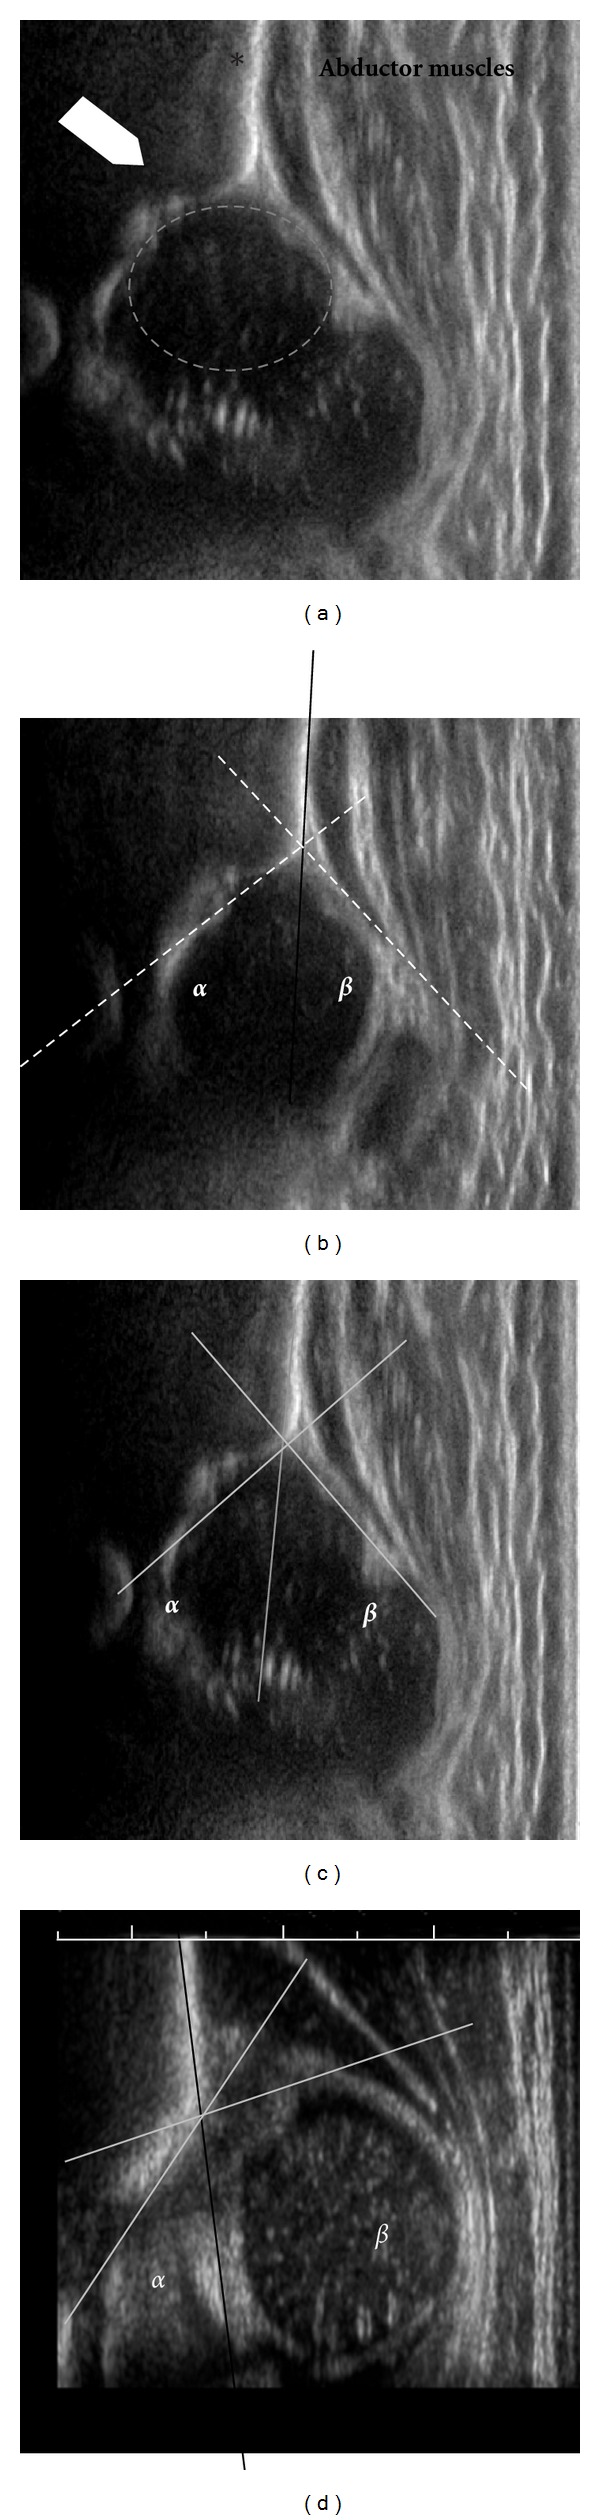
Ultrasound neonatal hip examination. (a) A representative longitudinal ultrasound image of a normal neonatal hip. The ilium is marked by the asterisk, the bony acetabular roof by the large arrowhead, and the abductor muscles seen by the longitudinal white/gray alternating structures. (b) Measurement of the alpha (*α*) and beta (*β*) angles on ultrasound establish the Graf class. The baseline is first drawn and is the line along the ilium as it intersects the bony and cartilaginous portions of the acetabulum (solid black line). The *α* angle is the angle between the baseline and the roof of the bony acetabulum; the *β* angle is the angle between the baseline and the cartilaginous roof. (c) An example of a Graf IIc hip, with an *α* angle of 43° and a *β* angle of 49°. (d) An example of a Graf IV hip, irreducible dislocated hip, with an *α* angle of 42°. Typically *β* angles are not measured on dislocated hips, but in this example it would measure 99°.
